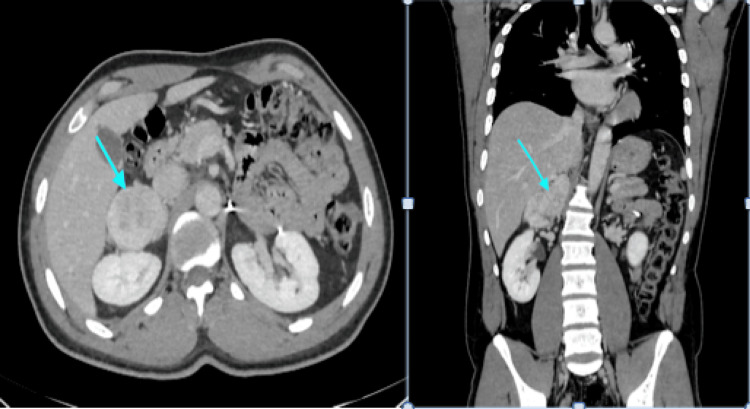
A coronal and transverse section of an abdominal CT scan showing a right adrenal tissue mass with lobulated contours, heterogeneously enhanced after contrast injection, measuring 76 × 54 × 34 mm in diameter CT: computed tomography
